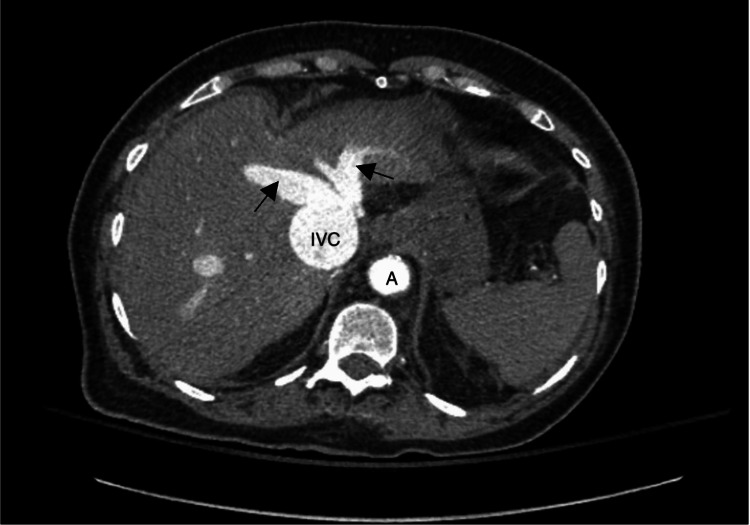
Repeat CT angiogram abdomen/pelvis: hepatic cut Evidence of inferior vena cava (IVC) dilation and contrast backflow into hepatic veins (arrows), consistent with hepatic congestion and elevated right atrial pressure (RAP). A: Aorta.
